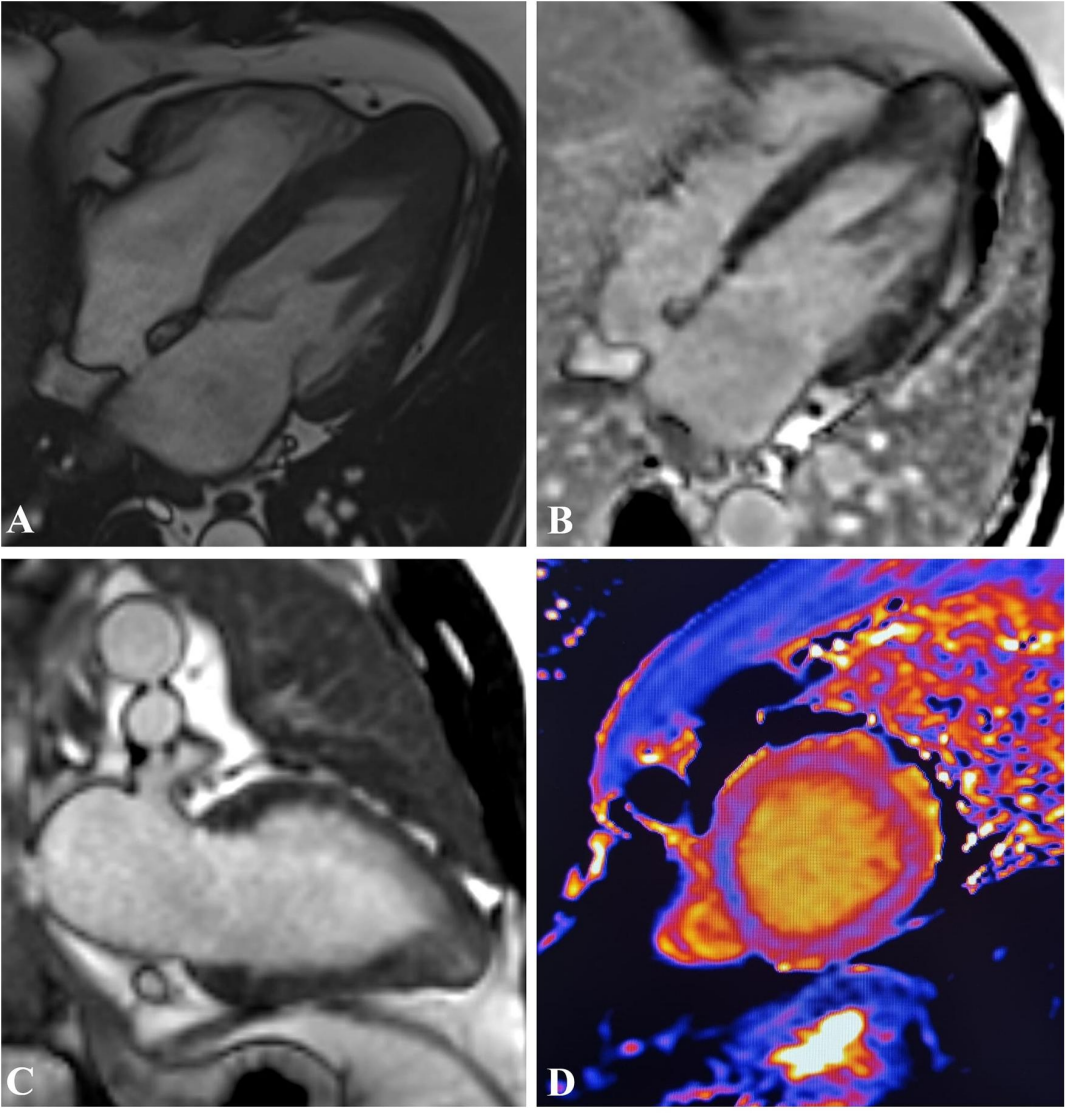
A 47-year-old patient with obstructive septal hypertrophy. The short-axis (A), three-chamber (B,D), and four-chamber (C) balanced free precession (b-SSFP) MRI sequence shows asymmetric septal thickening of the left ventricle with a maximum myocardial thickness of 24 mm and left ventricular outflow tract obstruction.
